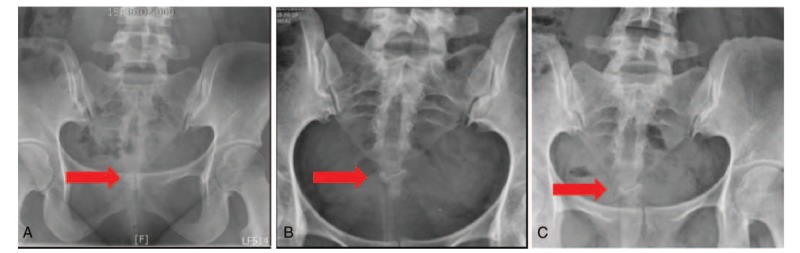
Anterior-posterior pelvic radiograph of the fracture at onset and post-therapy. (A) Image acquired immediately after the accident. Fracture dislocation of the sacrococcygeal joint (arrow) was noted after the traffic accident, which occurred on August 14, 2016. (B) Anterior-posterior pelvic radiograph acquired after 11 weeks of treatment, on March 1, 2017. The radiograph shows coccygeal healing (arrow). (C) Anterior-posterior pelvic radiograph acquired after 24 weeks of treatment, on May 31, 2017, shows coccygeal healing (arrow).
